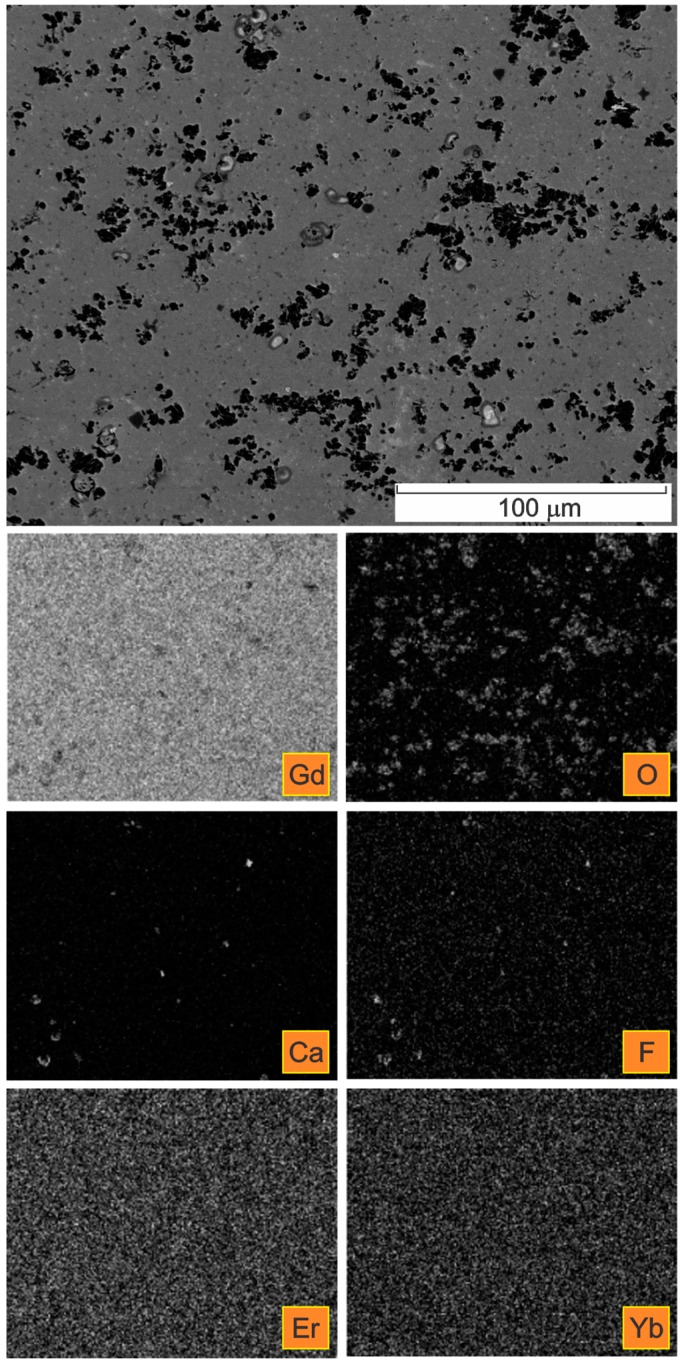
SE image of the microstructure in the centre of a Gd cylinder (extrusion transversal direction) with the corresponding X-ray elemental mappings.
